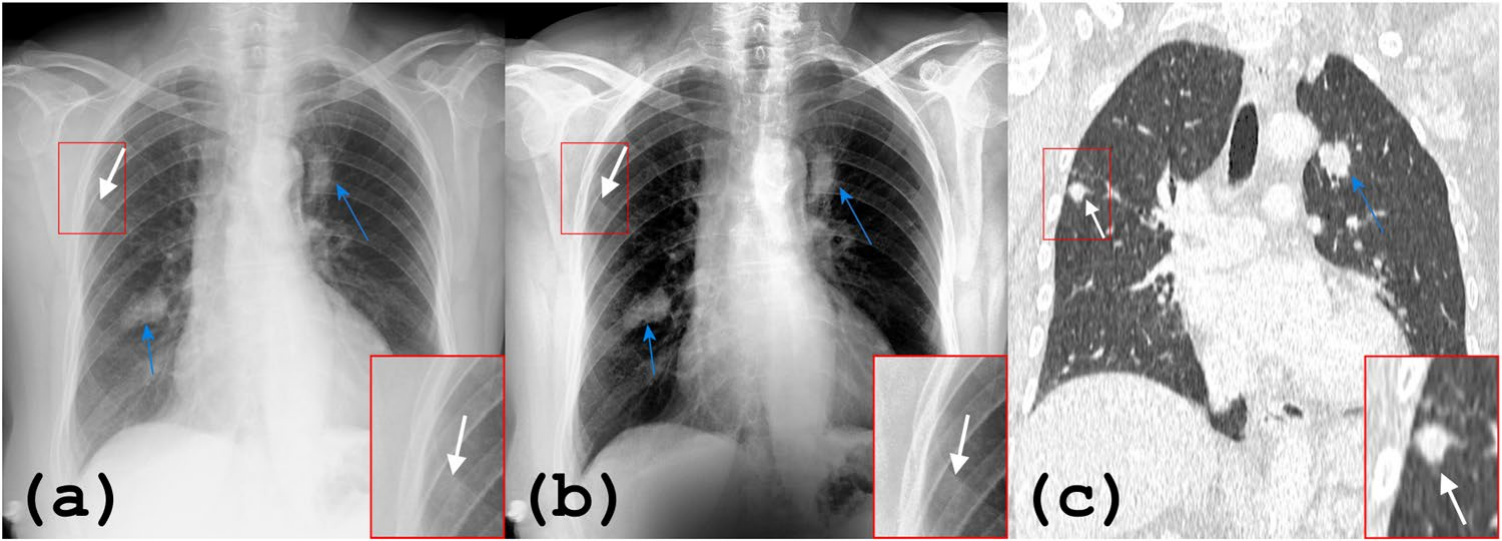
Chest radiography (a), resulting output using PACE2.0 (b) and (c) coronal multiplanar reconstruction of high-resolution computed tomography (CT) of a female patient with high-grade breast cancer. (a) The CXR reveals two metastases: one in the left upper lobe (indicated by a blue arrow) and one in the middle lobe of the right lung (also indicated by a blue arrow). However, the PACE2.0-enhanced image (b) reveals an additional small metastasis in the right upper lobe, which was previously obscured in (a) by a rib (indicated by a white arrow). The coronal CT (c) scan reconstruction (different plane) conclusively confirms the presence of the small lesion.
